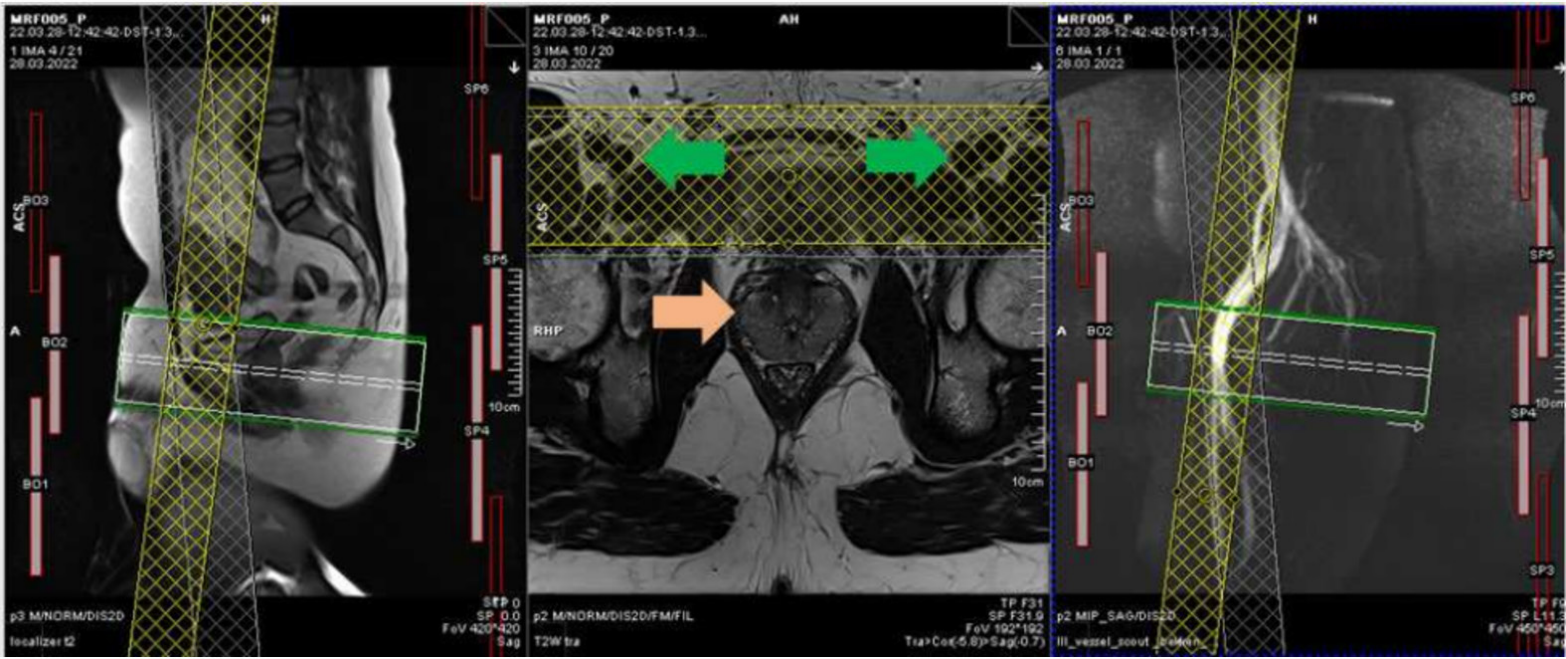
Screenshot from the MRI scanning computer with sagittal localiser (left), *T*
